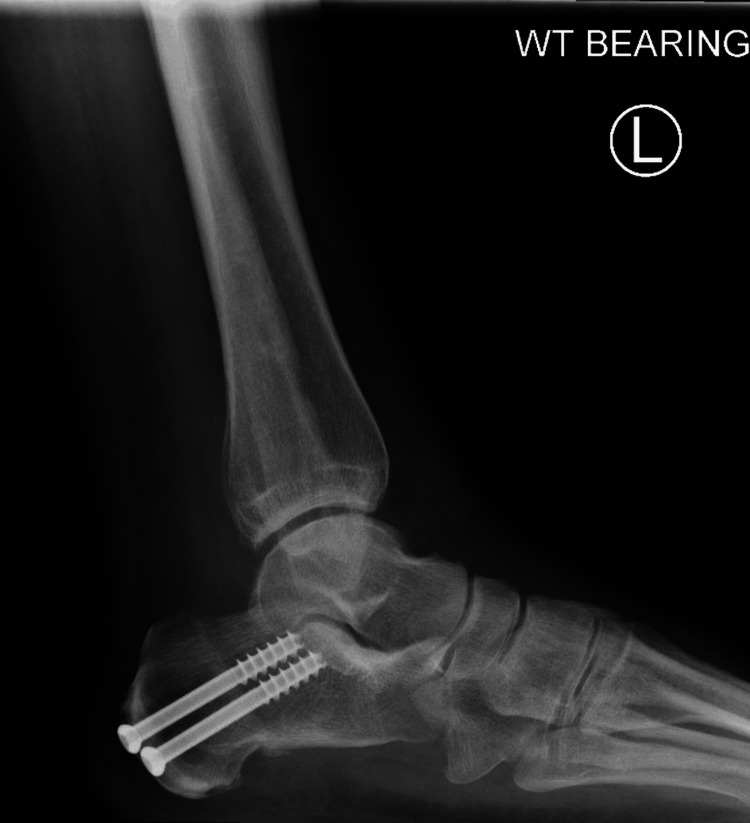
Final lateral radiograph showing no bony tunnel around the medial malleolus and reconstruction of the arch of the foot
